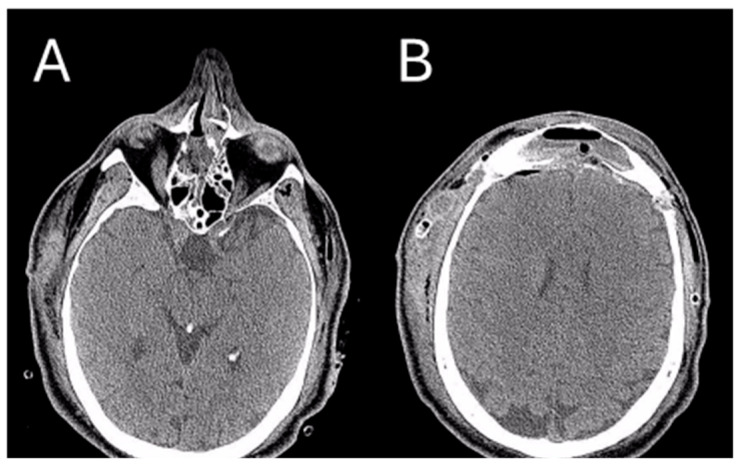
Post-operative CT image of Patient 2. Two axial cuts of the post-operative CT scan are appreciatedat the same level as those shown in Figure 3 to demonstrate the outcomes of the surgical removal. (A) the absence of the lesion localized at the ethmoidal level (B) The absence of the lesion localized in the right frontal sinus.
